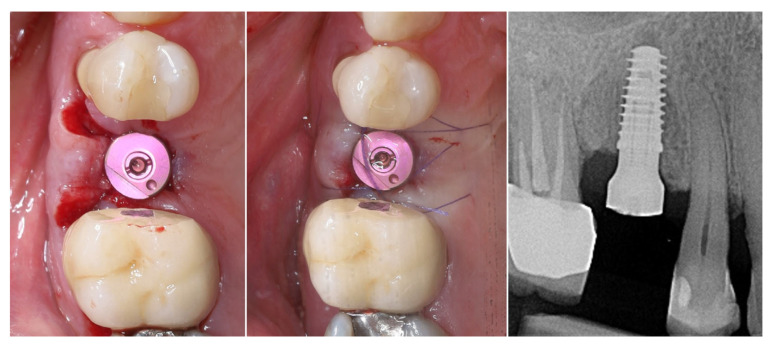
Clinical and X-ray view after implant placement and roll flap adaptation.
